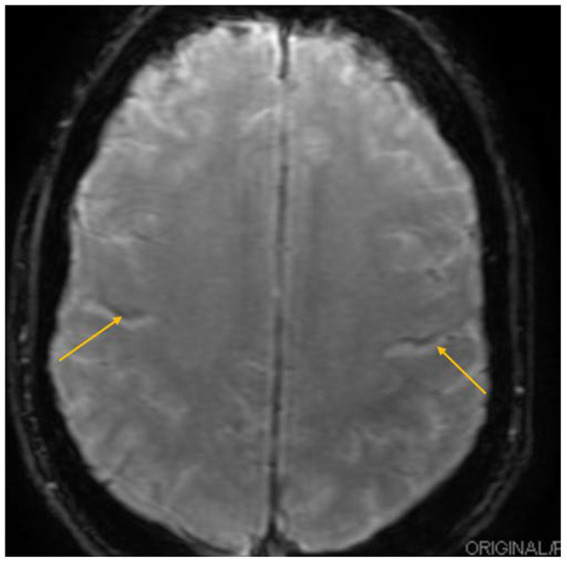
Brain MRI. MRI SWAN sequence showed motor band sign (arrows).
